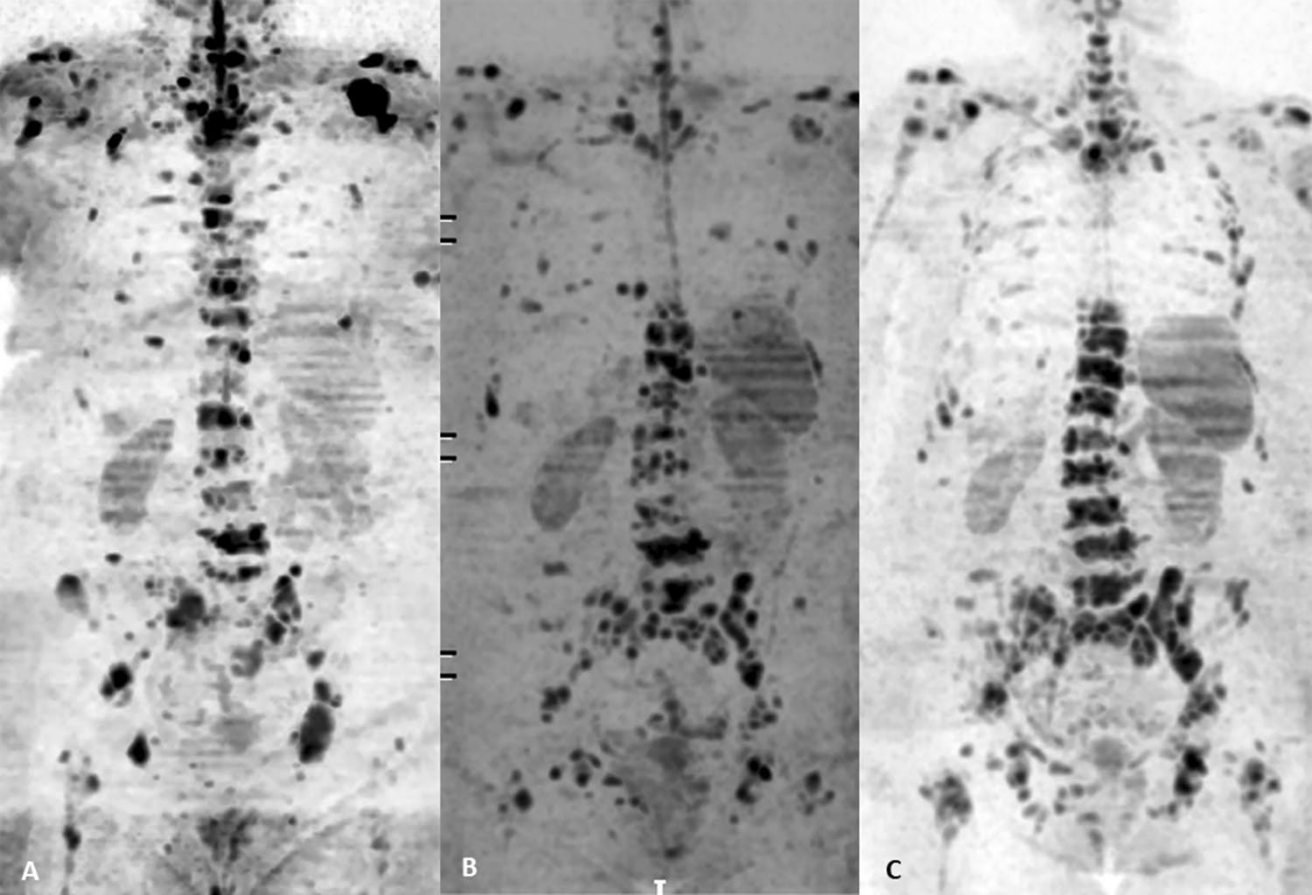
A 77-year-old patient with breast cancer presented with diffuse bone metastatic involvement in the initial staging with diffusion whole-body MRI (A, left) with a heterogeneous response at 6 months (B, center) given progression of pelvic, lumbar, and lower dorsal and costal bone disease and partial response of metastatic disease in middle and upper dorsal bodies and shoulder girdle. At the 3-month follow-up (C, right), we found a marked progression of bone disease of the generalized form
